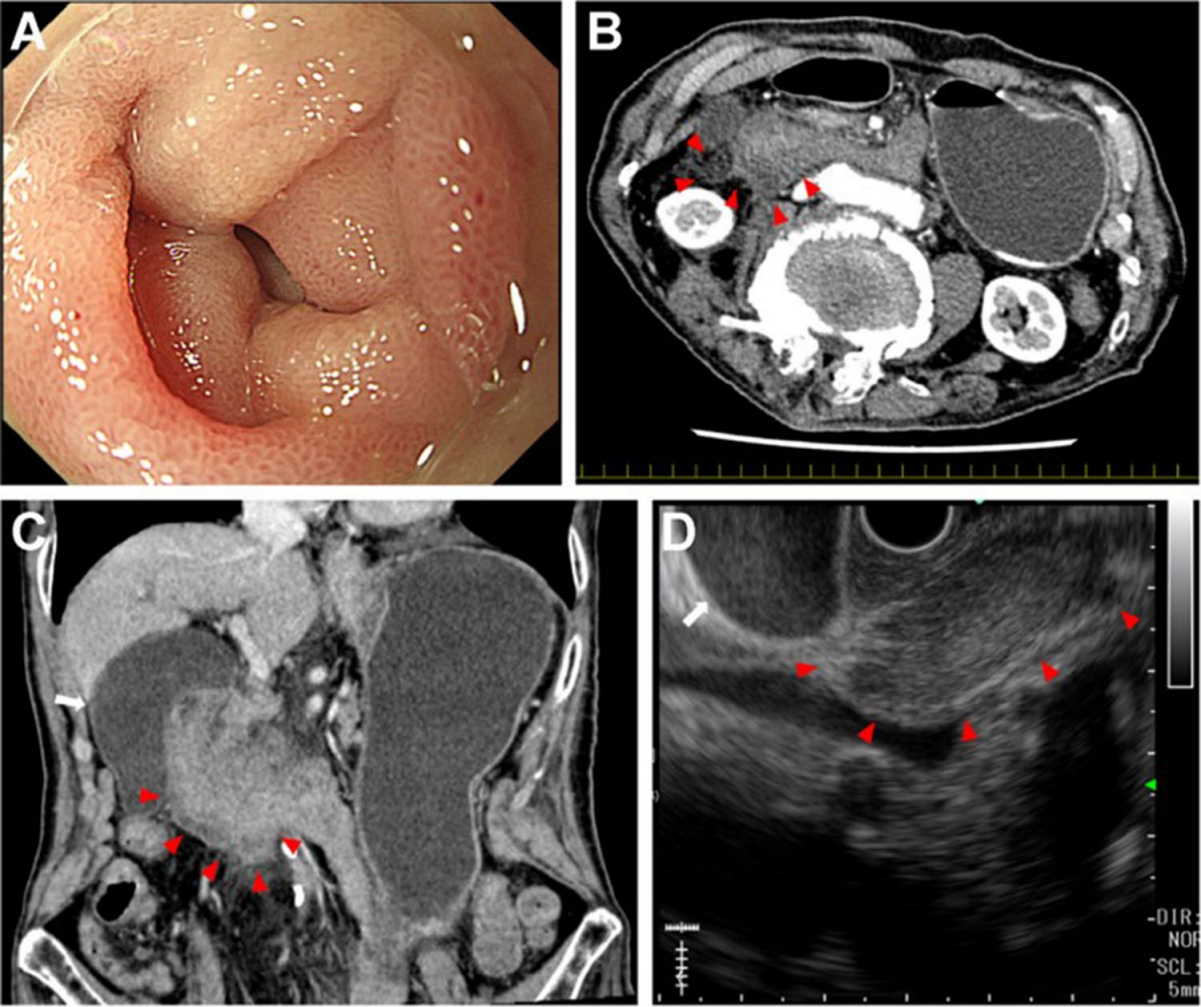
An 83- year-old male presenting with fever and vomiting (Case 2). a The EGD on day 3 showed edematous, reddish mucosa and narrowed lumen of the 2nd part of the duodenum. The endoscope did not pass through the lumen. b A dynamic contrast-enhanced CT on day 4 revealed a band-shaped low-density area spreading from the paraduodenal space to the right anterior pararenal extraperitoneal space (arrowheads) and appears to be in contact with the thickened wall of the 2nd and 3rd part of the duodenum. c In coronal reformatted images, the low-density area is located on the caudal side of the 2nd to 3rd part of the duodenum and obstructs it (arrowheads). There was a distention of the stomach and an enlargement of the gallbladder (white arrow). d EUS on day 11 revealed a 36-mm-sized oval-shaped low echoic mass (arrowheads) just outside of the thickened wall of the 2nd part of the duodenum. It extended along with the 3rd part of the duodenum in an L shape. A small amount of anechoic component and partially high echoic component were observed in the low echoic mass. An enlargement of the gall bladder (white arrow) was further identified
